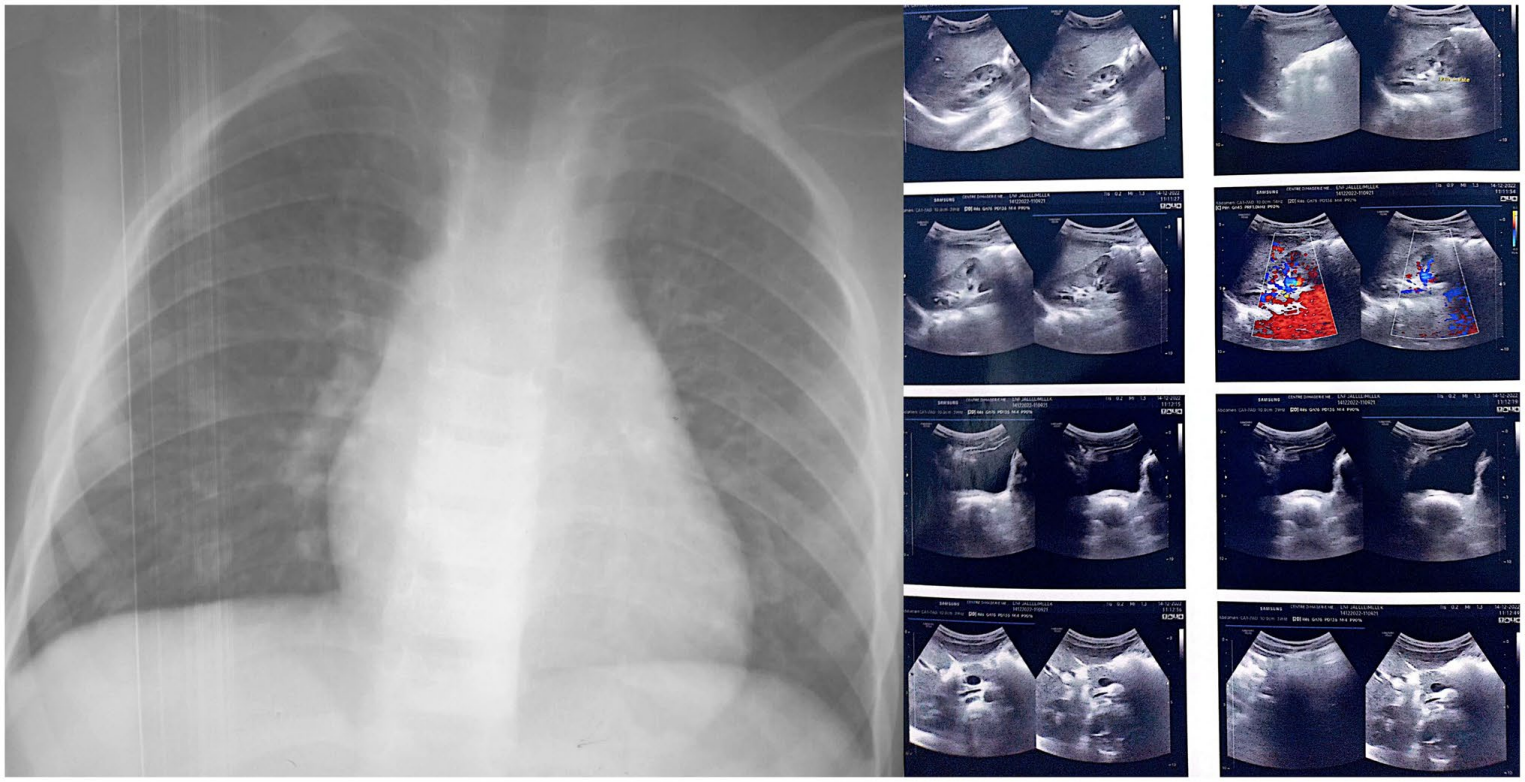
Posteroanterior chest x-ray (left image) and abdominal ultrasound (right image) showing no abnormalities
